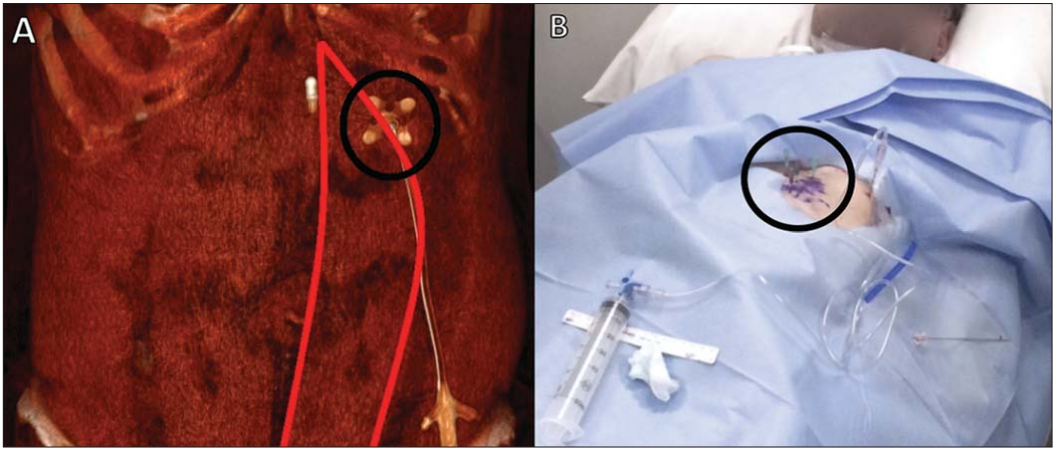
Marking the puncture site for CT-guided percutaneous gastrostomy.
